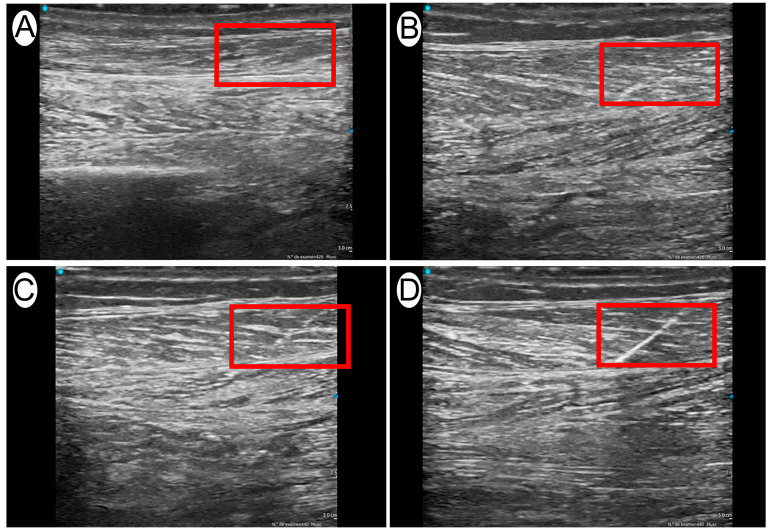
Representative ultrasound images of different PE doses applied for 1 s: (A) 0.10 mA; (B) 1.00 mA; (C) 2.00 mA; (D) 6.00 mA.
